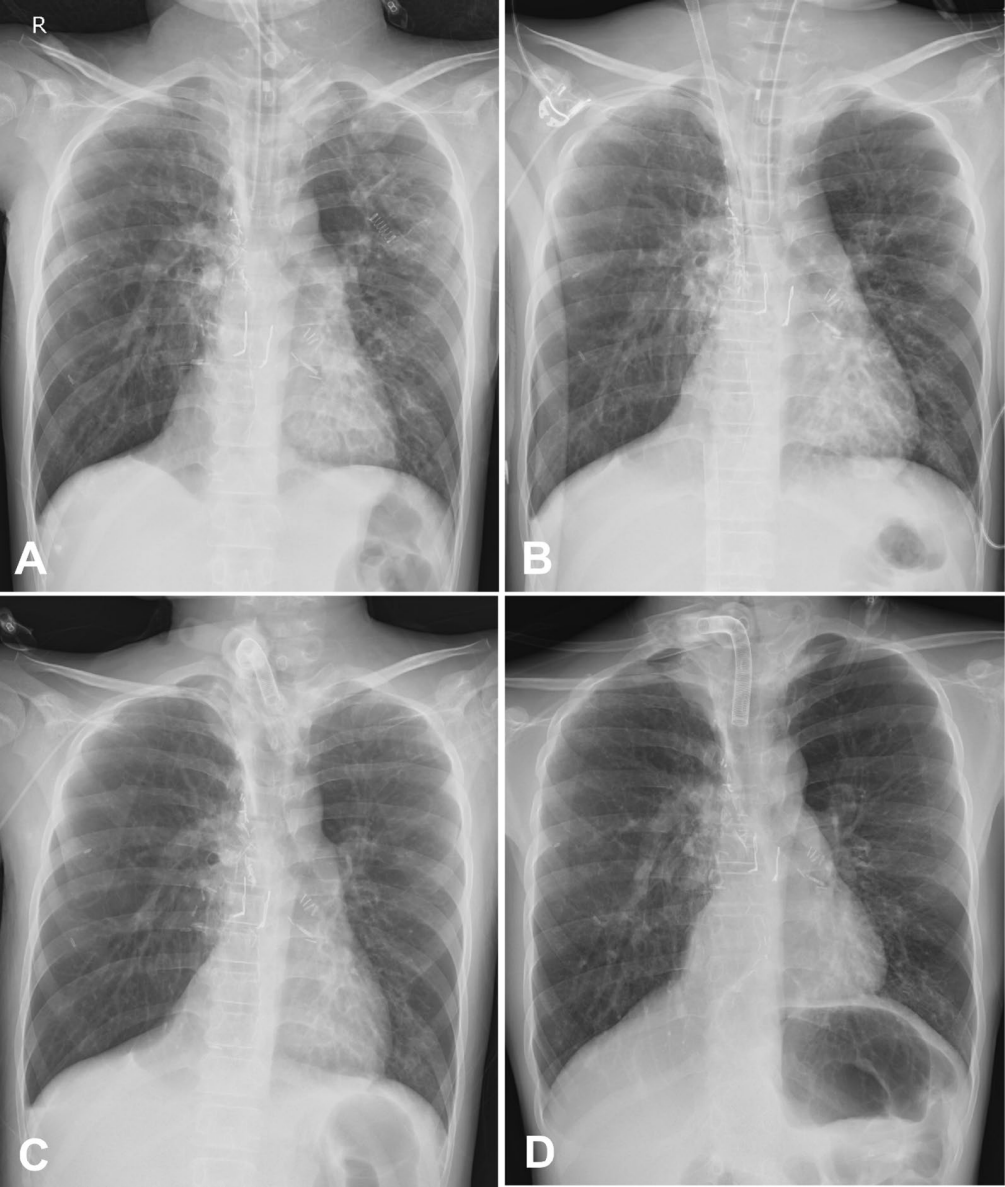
Chest X-ray images during the patient’s clinical course: A day 0 after ICU admission; B after ECMO initiation; C after removing ECMO; D before transfer. *ECMO* extracorporeal membrane oxygenation, *ICU* intensive care unit
